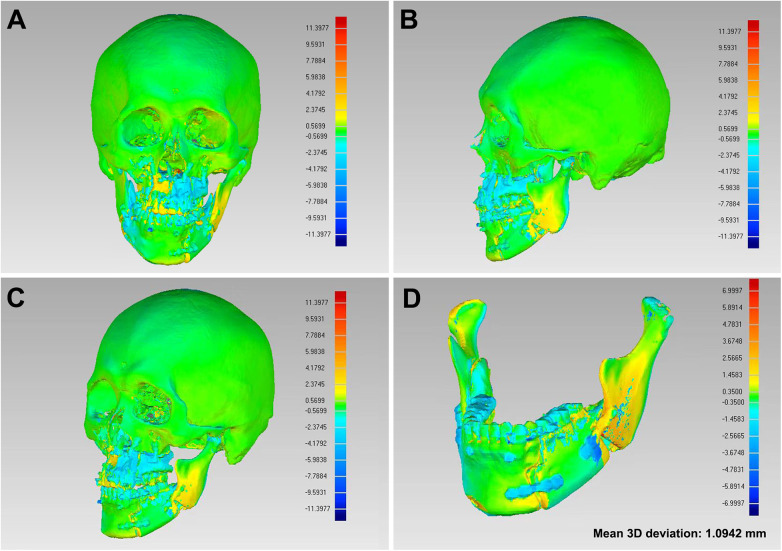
The 3D models of the virtual surgical plan and the CT scan of the actually achieved outcome were reconstructed to validate the surgical accuracy. (A) Frontal view; (B) lateral view; (C) oblique view; (D) detailed view of the mandibular body osteotomized area and mean 3D deviation of the mandible.
